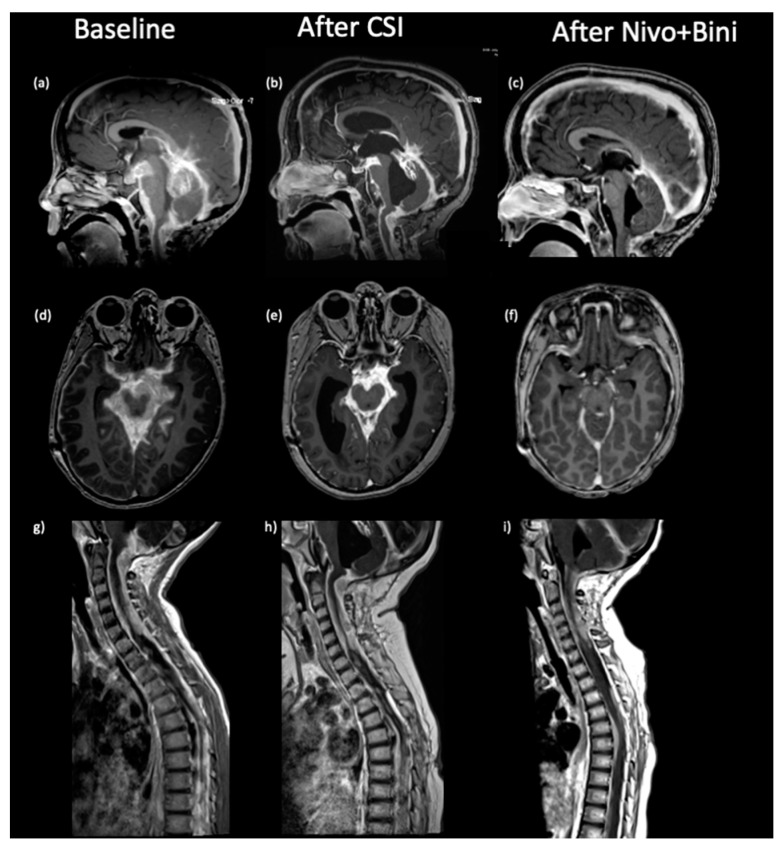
Sagittal (a–c) and axial (d–f) contrast-enhanced T1-weighted images of the brain and upper spine (g–i). At baseline, the brain demonstrated extensive enhancing leptomeningeal tumor with associated mass effect (a,d), as well as diffuse spinal leptomeningeal tumor, compressing the cord (g). Follow-up scans demonstrate decreased leptomeningeal enhancement in the basal cisterns (b,c,e,f) with relatively stable disease in the spine (h,i).
